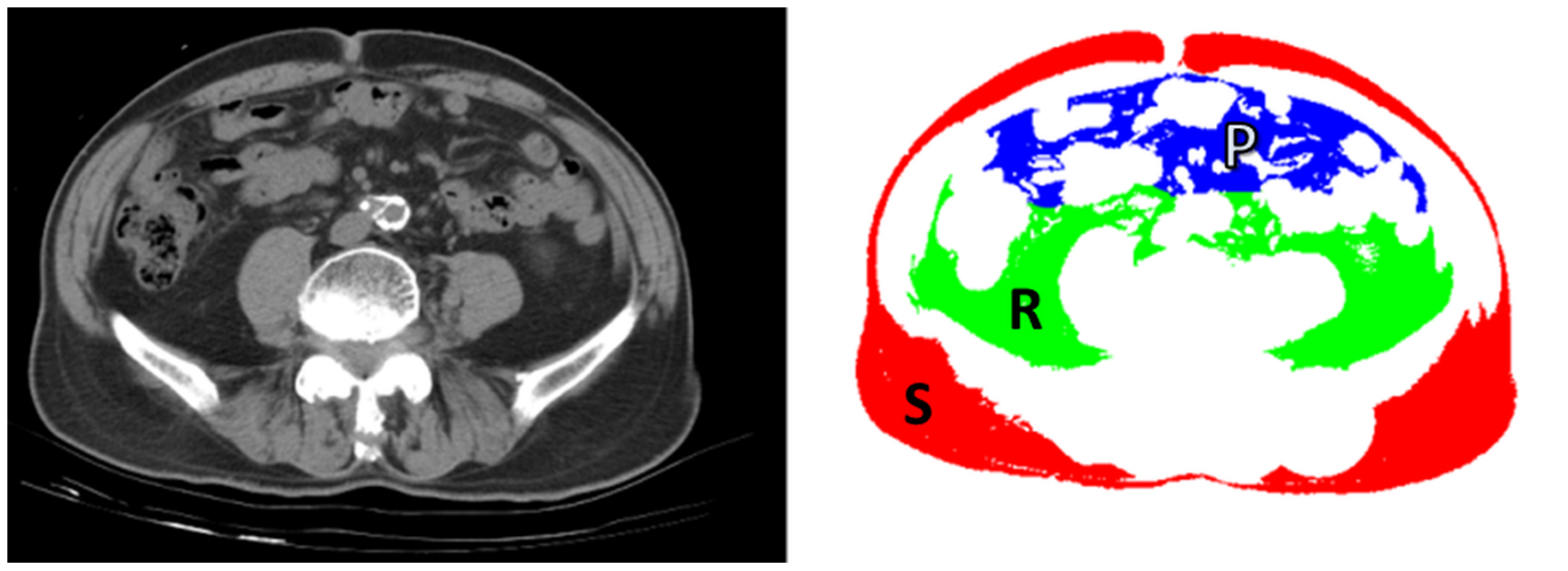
Image demonstration of determining abdominal fat distribution on a CT scan. Left, sample CT image obtained at the umbilicus level. Right, fat masks created for determining areas of subcutaneous fat (red, “S”), peritoneal fat (blue, “P”) and retroperitoneal fat (green, “R”) using methods described in the Materials and Methods section.
